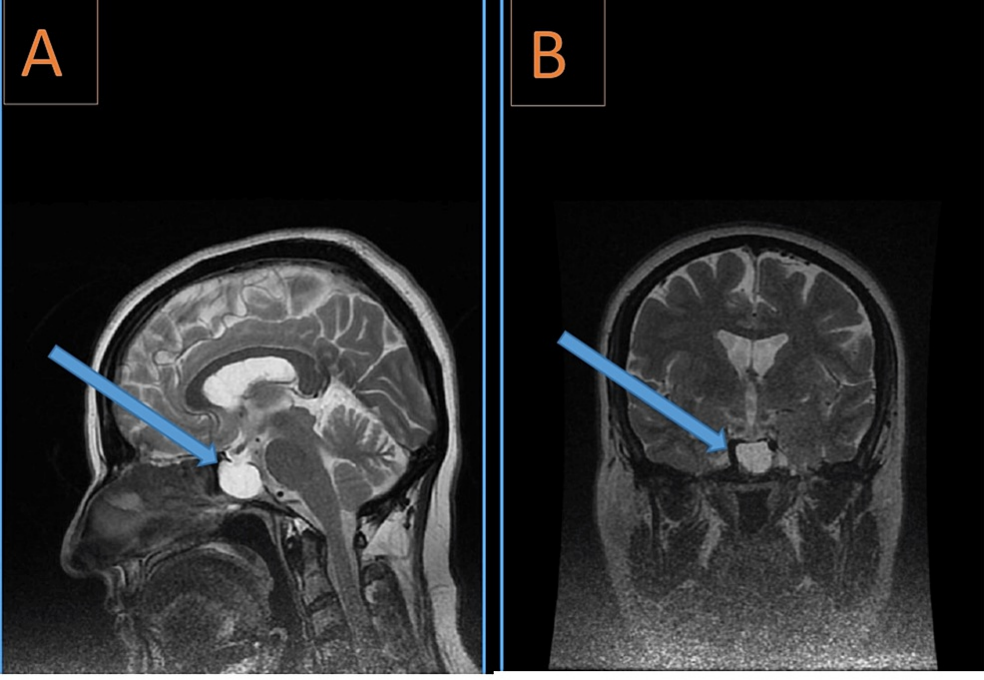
Magnetic resonance imaging of the brain Enlargement of sella turcica with empty sella on the (A) sagittal and (B) coronal views.
